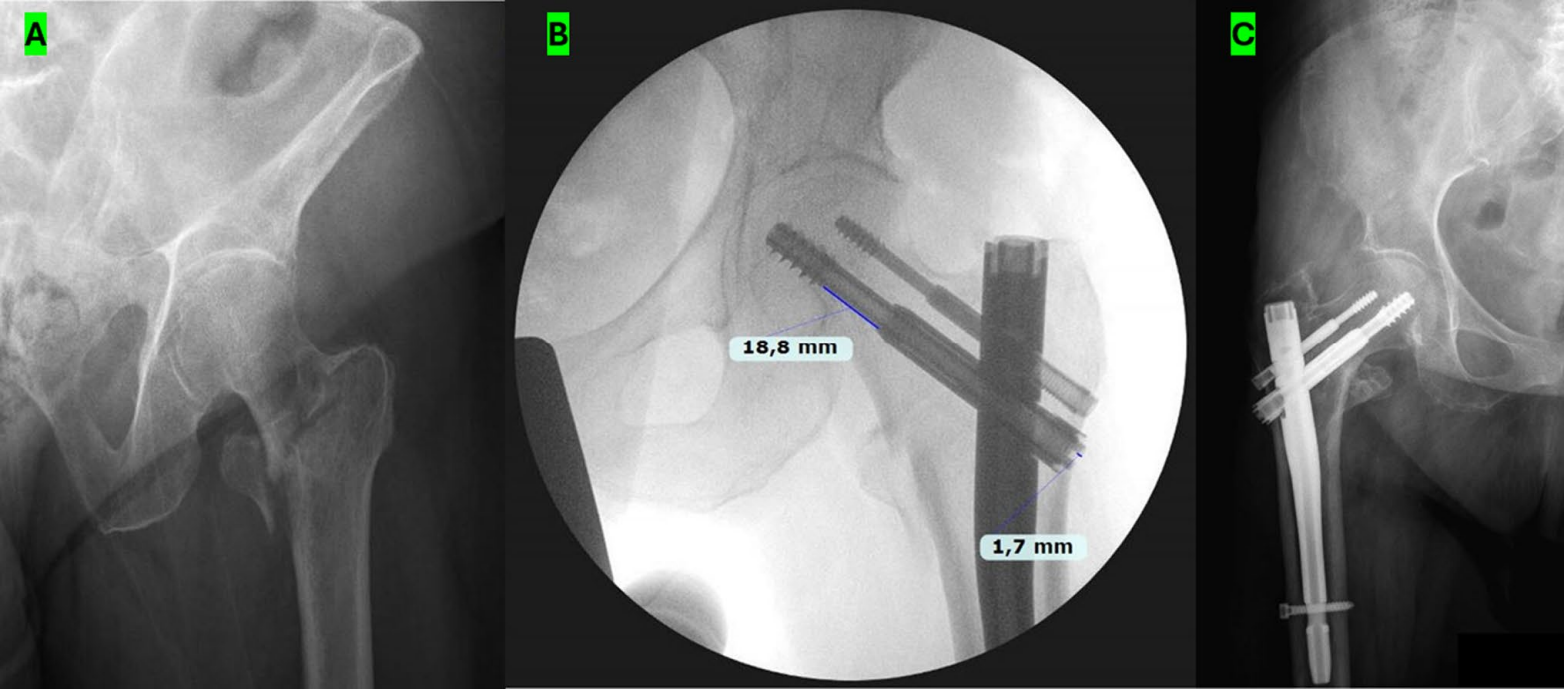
Chimaera trochanteric nail (Orthofix®, Lewisville, Texas, USA) comes in a 180 mm length and it is suitable for both, left and right femur. The proximal locking holes allow the placement of a lag screw and a second supplementary screw to provide additional rotational stability. The lag screw connects to the nail by a specifically designed crown that is part of the screw. A AO-OTA 31-A2.2 pertrochanteric fracture with detachment of the lesser trochanter. B The TAD distance is calculated by the sum of the distances from the tip of the lag screw to the apex of the femoral head on AP and LL radiograph after adjusting for magnification. Goal TAD is < 25 mm. C Plain AP radiograph showing the femoral bone healed after a pertrochanteric fracture treated with CRIF. Bone healing process after intramedullary nail fixation occurs by endochondral ossification. The shortening of the lag screws and the lateral protrusion of the sliding screws calculated on the AP projection. This feature of the nail is intended to minimize the risk of screw cut-out, by providing immediate linear compression and allowing safe weight bearing during the process of fracture consolidation
